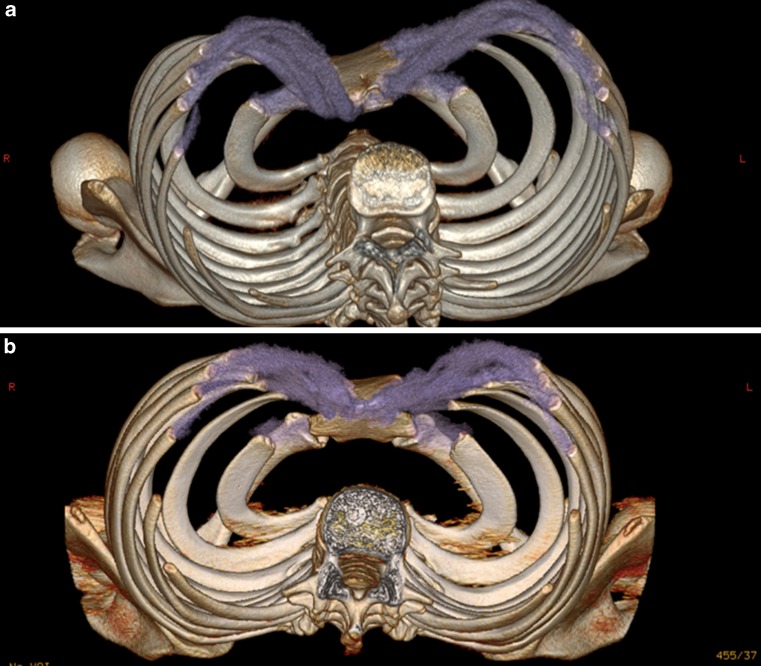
a Preoperative CT imaging of a 15-year-old female with an asymmetrical funnel chest. b Preoperative CT imaging of a 16-year-old boy with an asymmetric deep funnel chest
